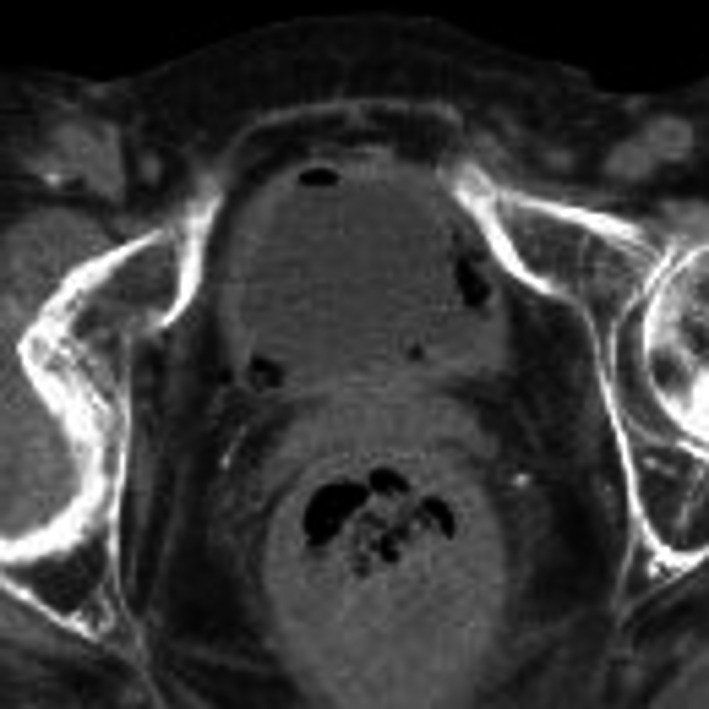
A computed tomography scan showing gases within the bladder and bladder wall
